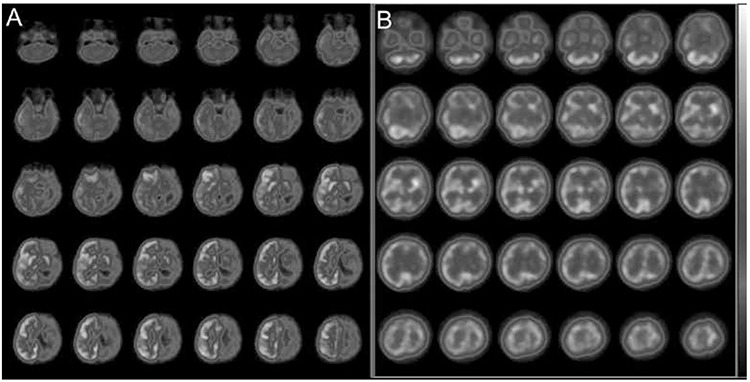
Interictal brain fluorine-18 fluorodeoxyglucose positron emission tomography/computed tomography of a patient with Rasmussen’s syndrome showed extensive hypometabolism in the left cerebral hemisphere involving the ipsilateral basal ganglion and the thalamic nucleus (A). On the ictal Tc-99m hexamethyl propylene amine oxime perfusion single-photon emission computed tomography diffuse increased perfusion in the corresponding regions was observed indicating the distribution of seizure activity (B)
